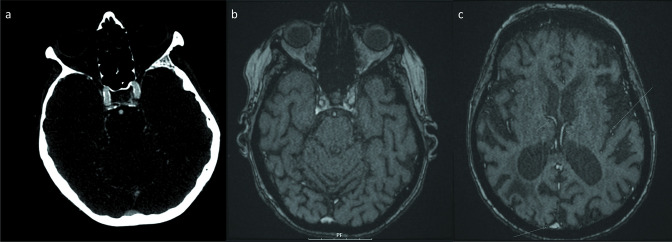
(a) CTA of the head demonstrates cavernous right internal carotid artery calcifications. (b) On MRA of the head, these cavernous right internal artery calcifications are not seen. (c) More superior MRA head image shows hypointense signal along the surface of the distal left middle cerebral artery branches, internal cerebral veins, and the superior sagittal sinus (arrows) due to presence of intravascular feuromoxytol. CTA, CT angiography; MRA, MR angiography.
